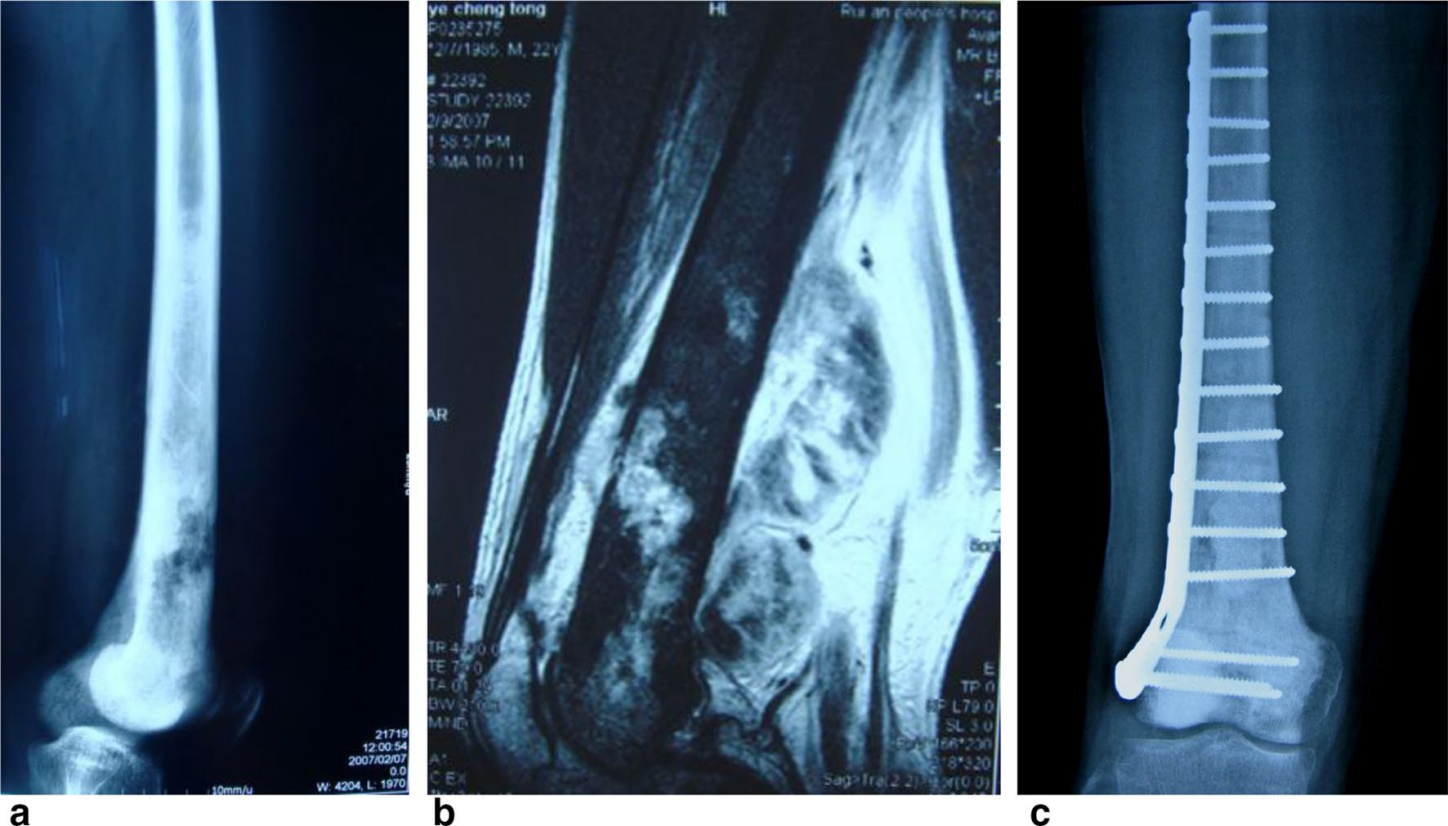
A patient with osteosarcoma involving nearly 3/4 length of the femur. The function is excellent after surgery for more than 10 years. a X-ray film showing the lesion involved the majorlity of the femur, b MRI showing the huge tumor bulk, c X-ray film post-operation, the function is perfect at 10 years after surgery
